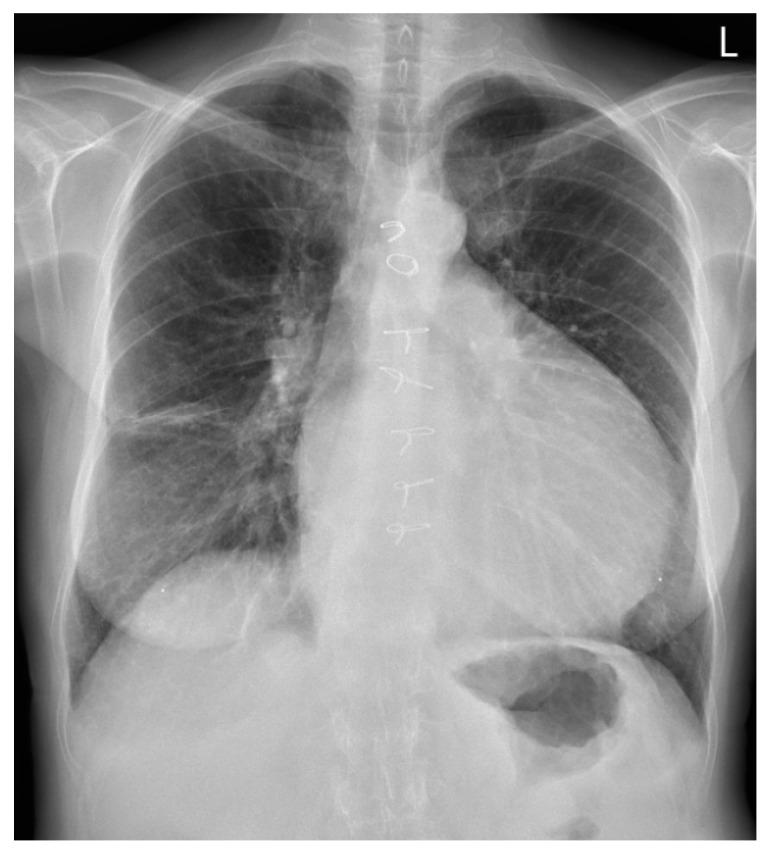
Posteroanterior chest X-ray showing marked cardiomegaly, predominantly due to the pseudoaneurysm.
